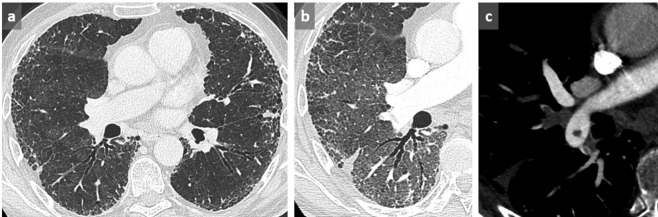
Pulmonary embolism in a patient with suspected acute exacerbation of interstitial lung disease. 73-year-old male known for drug-induced pulmonary fibrosis (a) (chemotherapy for high-grade bladder carcinoma), presenting with increasing dyspnea, fever and oxygen desaturation. Although a new GGO was observed and attributed to fibrosis exacerbation (b), pulmonary emboli were detected on this contrast-enhanced chest CT (c). Note the triangular opacity corresponding to a small intrafissural effusion in (b). This example highlights the importance of performing a contrast-enhanced CT in case of clinical worsening in patients with pulmonary fibrosis to rule out pulmonary embolism, since acute exacerbation is a diagnosis of exclusion. GGO, ground glass opacity.
